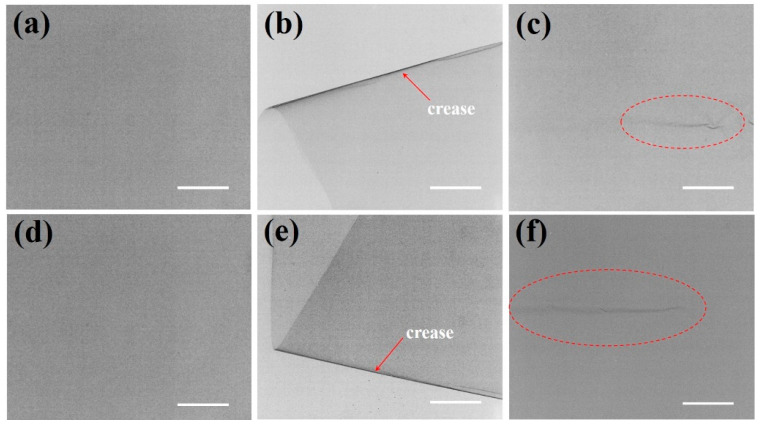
X-ray computed tomography images of different states between AF and MF samples. MF: (a) Initial state, (b) bending process, (c) final hemming state. AF: (d) Initial state, (e) bending process, (f) final hemming state. The scale bar in the image is 5 mm.
